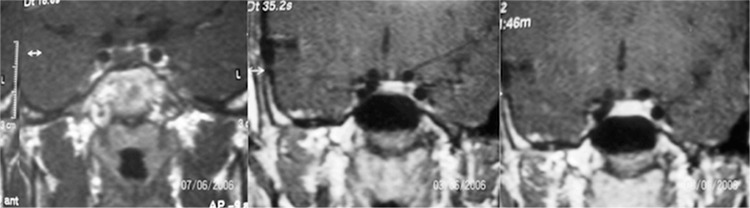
12x7 mm bilobulated hypointense lesion (hypophyseal adenoma), which is seen in 35. second in contrasted dynamic MRI, but not in 3 minute.
